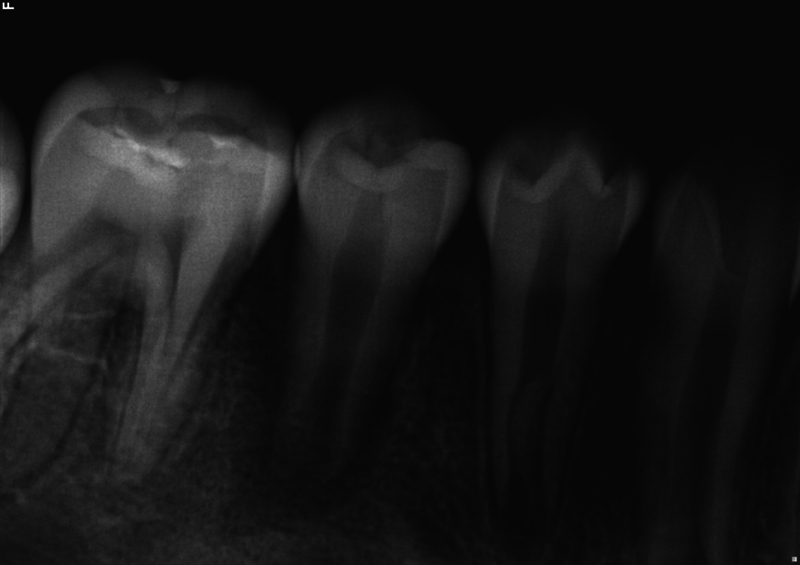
Preoperative periapical radiograph of tooth #29 taken at the University of North Carolina at Chapel Hill Adams School of Dentistry.
